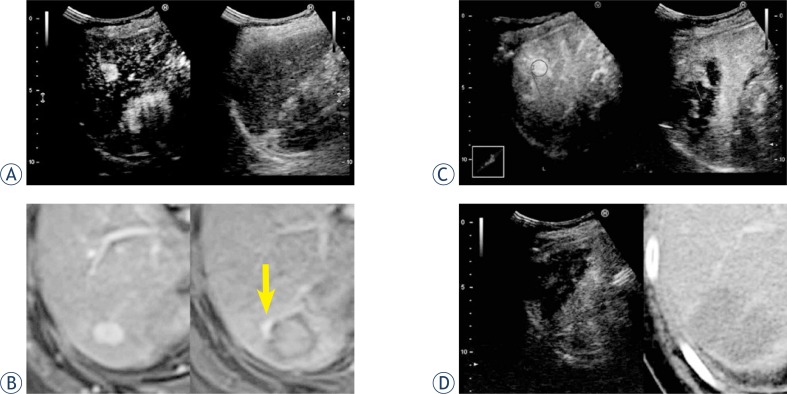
Usefulness of CEUS and RVS using 3DUS data in identifying HCC nodules requiring additional RFA. A 66-year-old female patient had a 13-mm HCC in segment 6. Because HCC was poorly visualized with conventional US, RFA was performed under CEUS guidance. After the first RFA session, dynamic MR showed a residual viable portion, but the second RFA session failed to ablate that portion. CEUS and RVS using 3DUS data clearly showed the portion requiring additional RFA. Therefore, a third RFA session was performed, which ablated the portion. A. CEUS before RFA. B. Dynamic MR imaging before RFA (left) and after the first RFA session (right). A residual viable portion is shown (arrow). C. CEUS and RVS using 3DUS data displays the portion requiring additional RFA (spherical marker). Each synchronized straight marker is drawn on the same posterior branch of the right branch of the portal vein. D. CEUS (left) and dynamic CT (right) suggest sufficient ablation. CEUS = contrast-enhanced ultrasonography; CT = computed tomography; HCC = hepatocellular carcinoma; MR = magnetic resonance; RFA = radiofrequency ablation; RVS = real-time virtual sonography; 3DUS, three-dimensional ultrasonography
